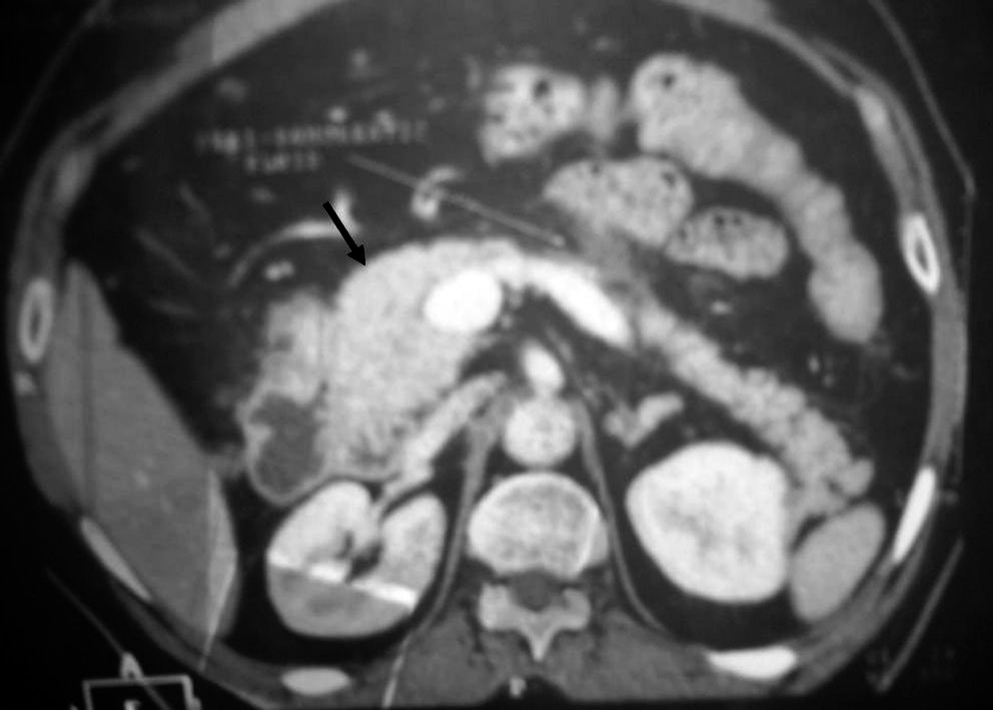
CT scan shows head of the inflamed pancreas
